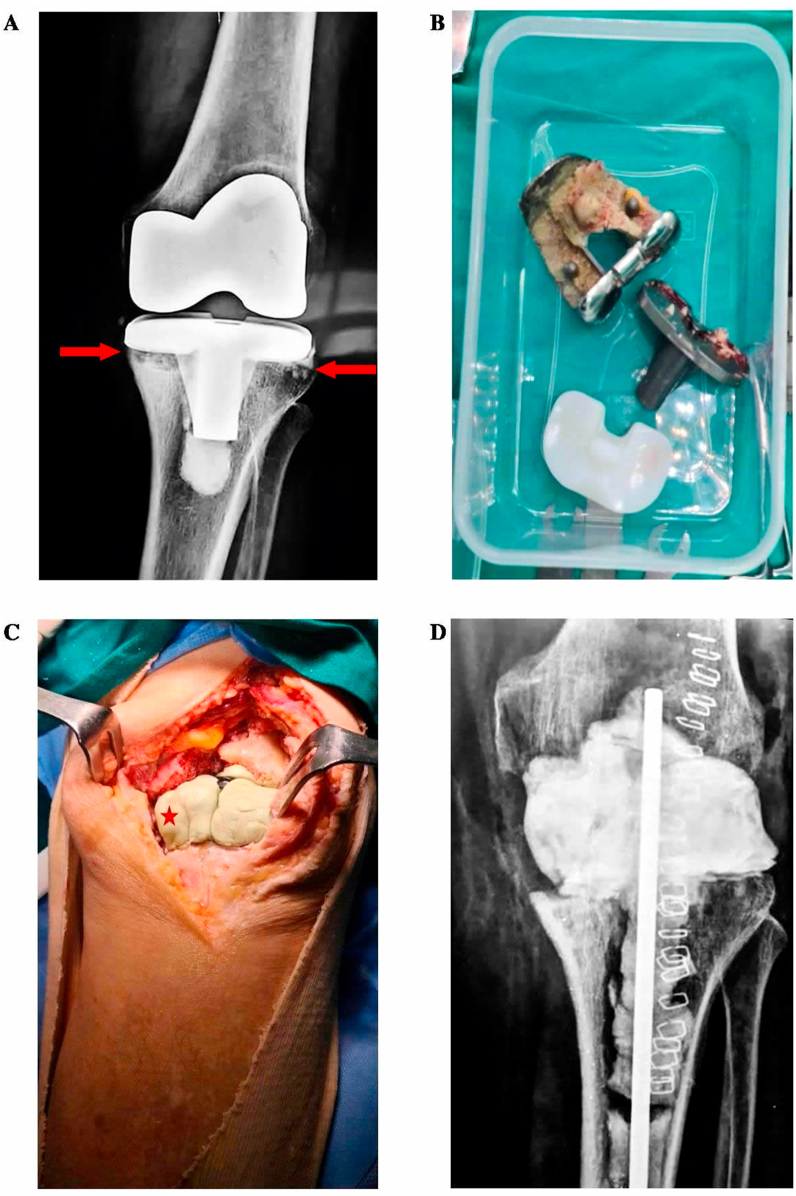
Collection of explanted prostheses in a two-stage TKA. (A) X-ray of a patient with loosening if his TKA. The arrows show the radiolucent appearance of loosening around the tibial prosthesis. (B) Explanted prostheses were transferred to the microbiology de? lkjbpartment for sonication in a sterile solid air-tight container. (C) The femoral and tibial tunnels were filled with vancomycin-impregnated cement beads until second stage of revision. The asterisk shows the cement filling the gap (D) The final x-ray after the first phase of revision. The patient received antibiotic therapy according to conventional and sonication fluid cultures. After a 2-year follow up his Oxford Score was found 29/60.
